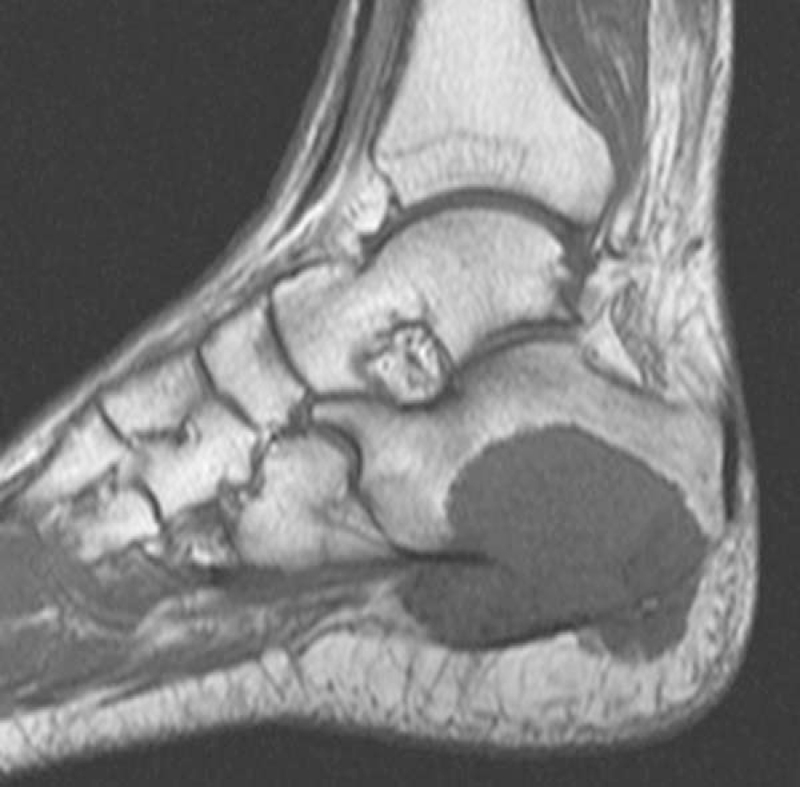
A sagittal T1-weighted MRI shows the area of decreased signal intensity area in the calcaneal body and the extraosseous mass. MRI = magnetic resonance imaging.
